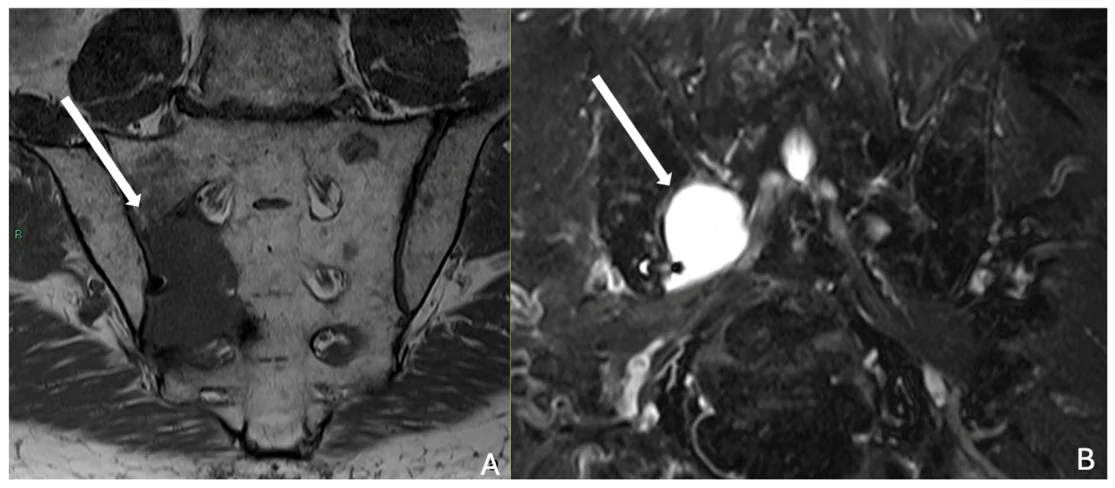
Case 5. A 68-year-old woman with known multiple myeloma presented with progressive right-sided lumbosacral pain, predominantly nocturnal, radiating to the right lower limb. Coronal T1-weighted pelvic MRI (A) demonstrates a focal marrow-replacing lesion within the right sacral ala (white arrow) . Axial fat-suppressed T2-weighted imaging (B) shows extraosseous soft-tissue extension involving the presacral region and adjacent sacral nerve roots of the lumbosacral plexus (white arrow). Original Image.
